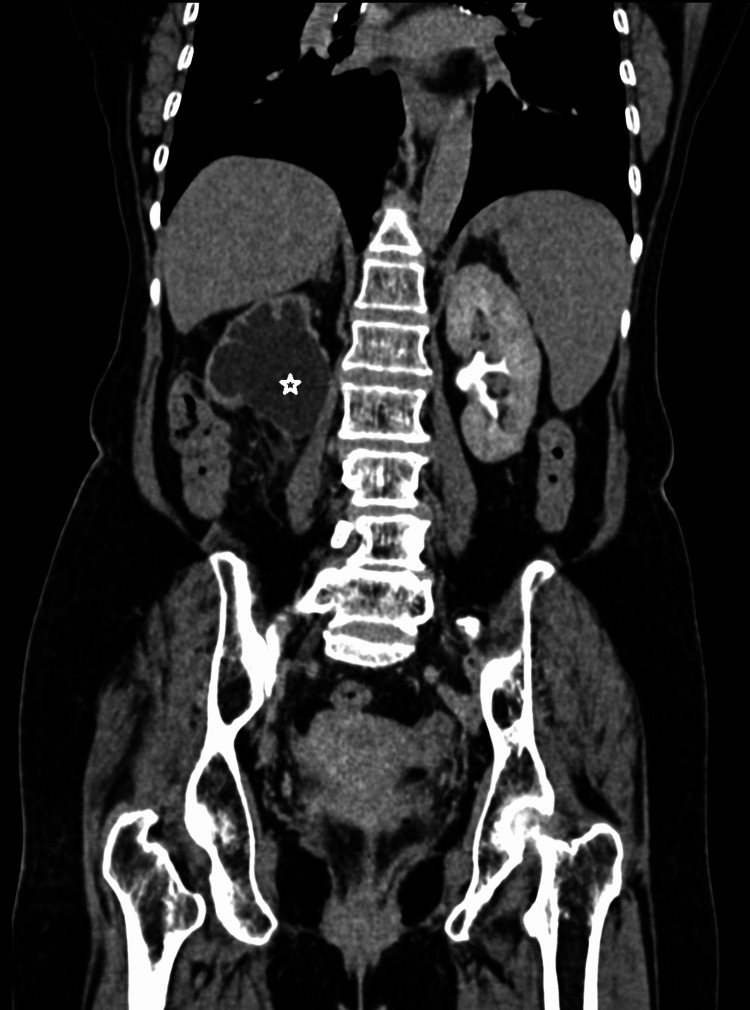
CT urography image in a coronal section of the excretory phase, showing no contrast excretion in the right kidney with upstream grade 4 hydroureteronephrosis (star) due to compression by mass lesion. The left kidney shows normal contrast excretion CT: computed tomography
